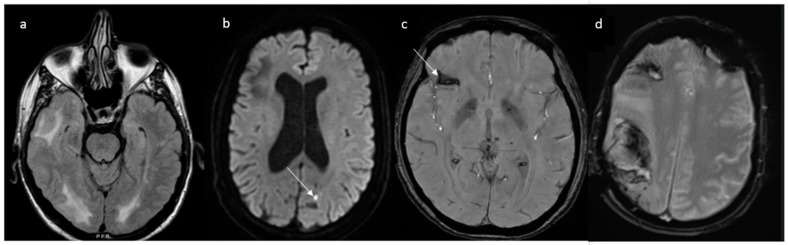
An overview of the potential complications of RCVS in four different patients. (a) Posterior reversible encephalopathy syndrome appearing as hyperintense lesions on fluid-attenuated inversion recovery imaging (FLAIR); (b) ischemic strokes (arrow) highlighted by diffusion-weighted imaging (DWI); (c) convexity subarachnoid hemorrhage shown as the hypointensity, demonstrated by gradient echo imaging that indicates the presence of blood within the cortical sulci; (d) temporo-parietal and frontal lobar parenchymal hemorrhages. RCVS: reversible cerebral vasoconstriction syndrome. FLAIR: fluid-attenuated inversion recovery. DWI: diffusion-weighted imaging.
